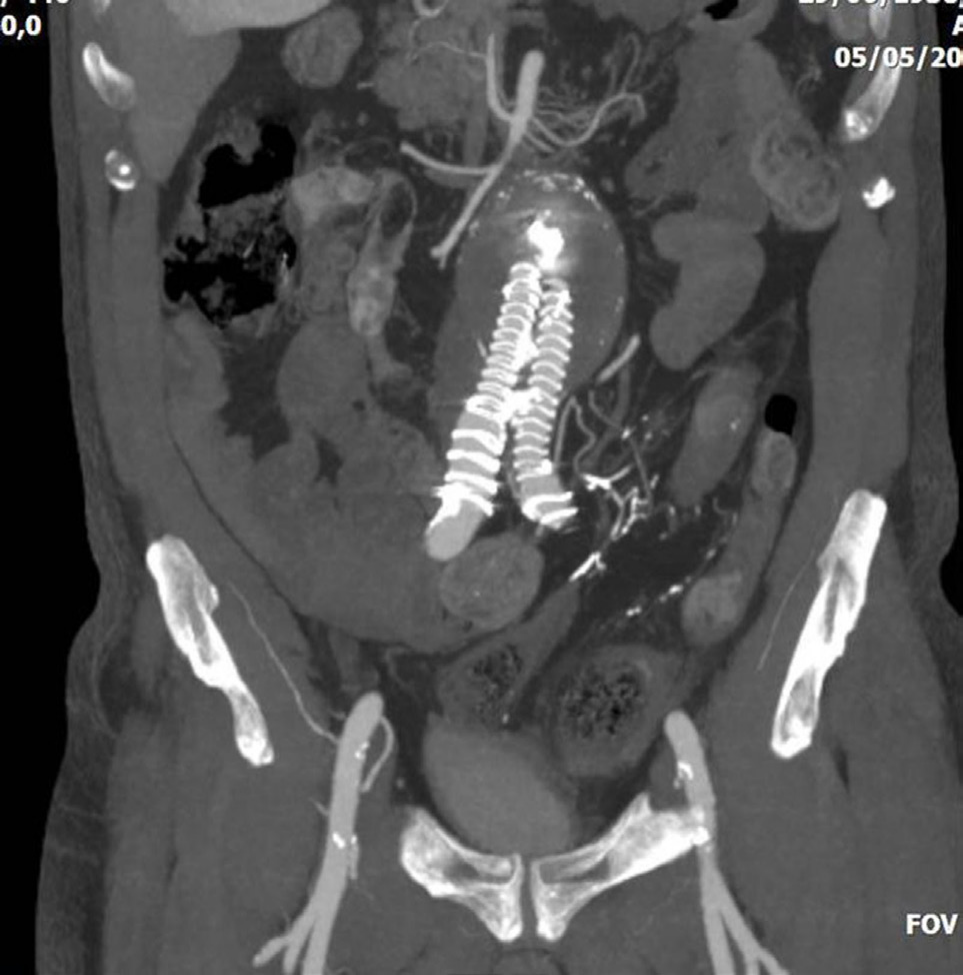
Abdominal CT of a 75-year-old patient who underwent endovascular abdominal aortic aneurysm repair with a covered aortic stent-graft. The patient had a type II endoleak *via* inferior mesenteric artery, and was treated with direct puncture and embolization with glue. Later, the patient presented severe colonic ischemia with sepsis. Embolic material is evident in the inferior mesenteric artery, whereas the sigmoid colon appears with thickened necrotic wall.
